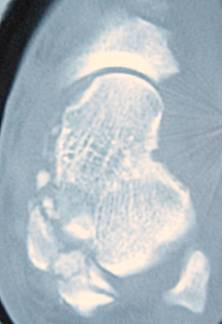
CT scan of the ankle.
